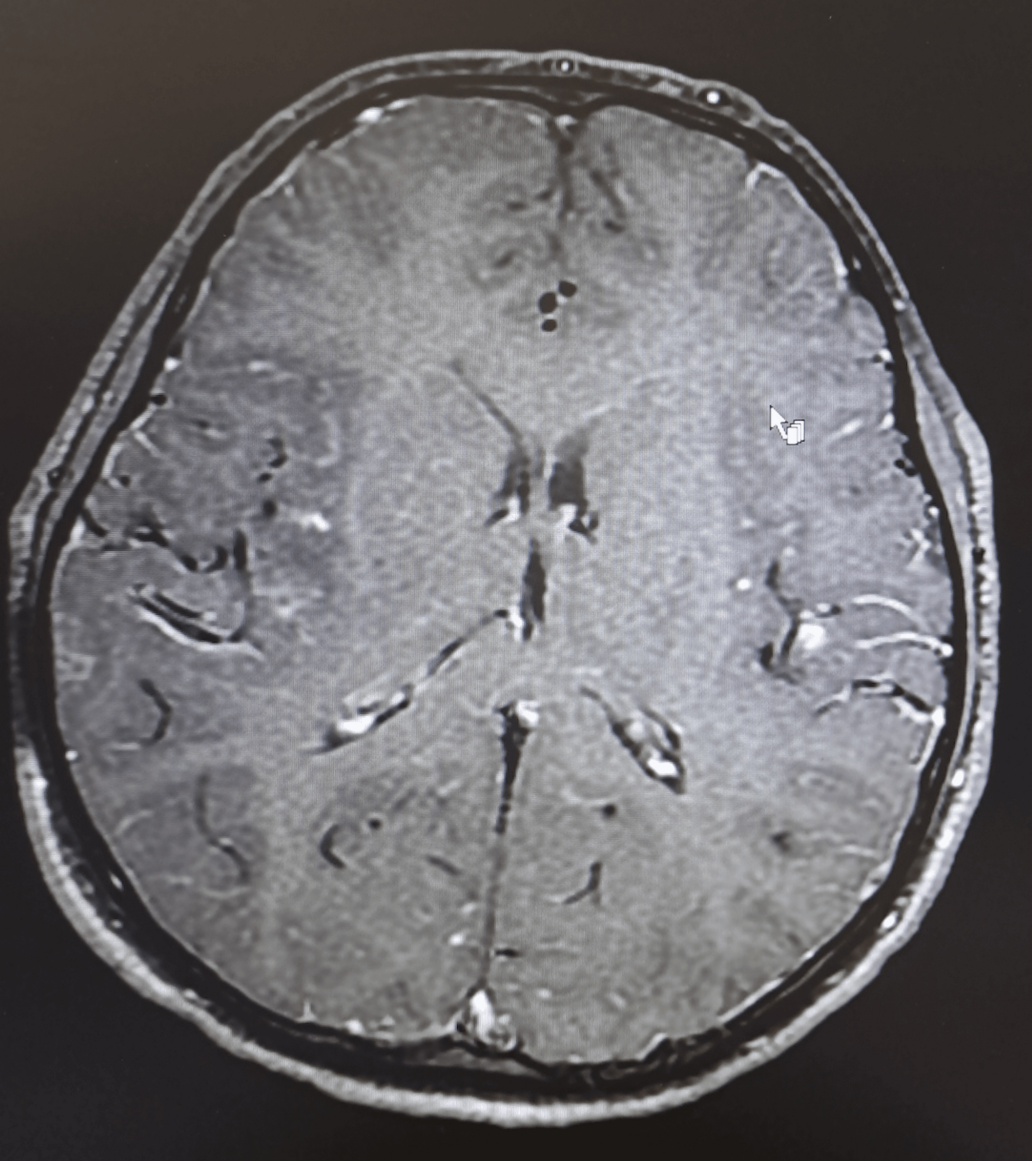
Axial T1-weighted post-contrast MRI of the brain upon admission. It reveals diffuse brain edema and subtle leptomeningeal enhancement over the insular cortex.
